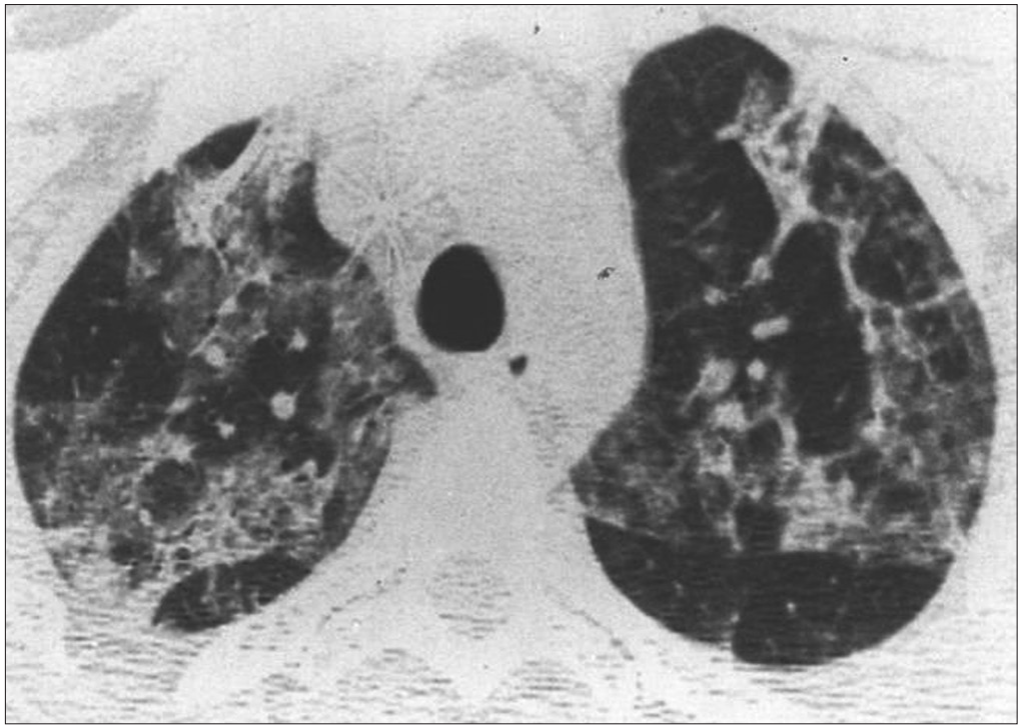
Pneumocystis carinii* pneumonia. Computed tomography (CT) in a subacute phase showing foci of consolidation and interlobular septal thickening due to organized inflammatory infiltrate on high-resolution CT
